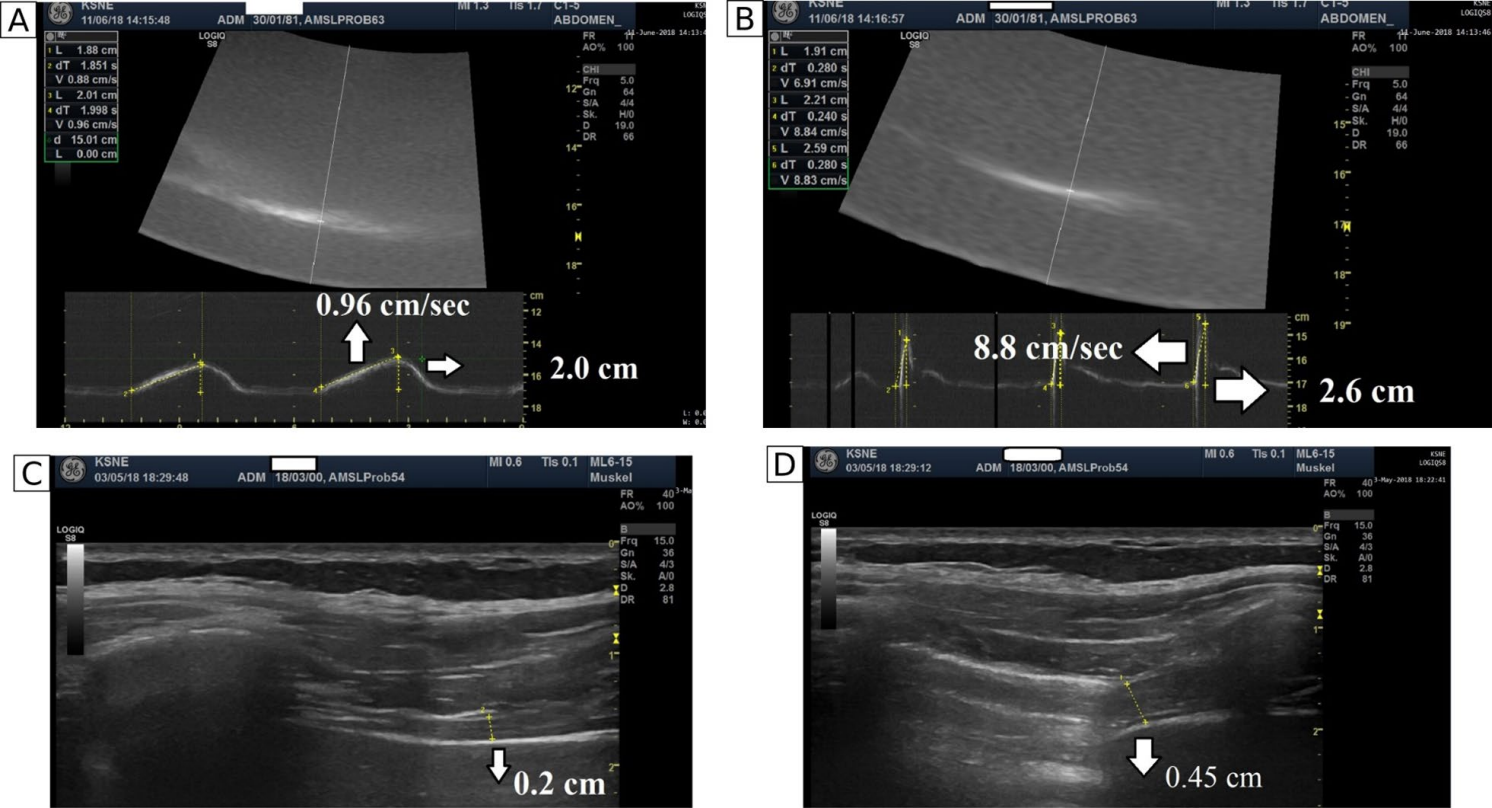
Parameters measured during diaphragm ultrasound: diaphragm excursion during tidal breathing (A) and sniff maneuver (B); and diaphragm thickness at functional residual capacity (FRC) (C) and at total lung capacity (TLC) (D)
